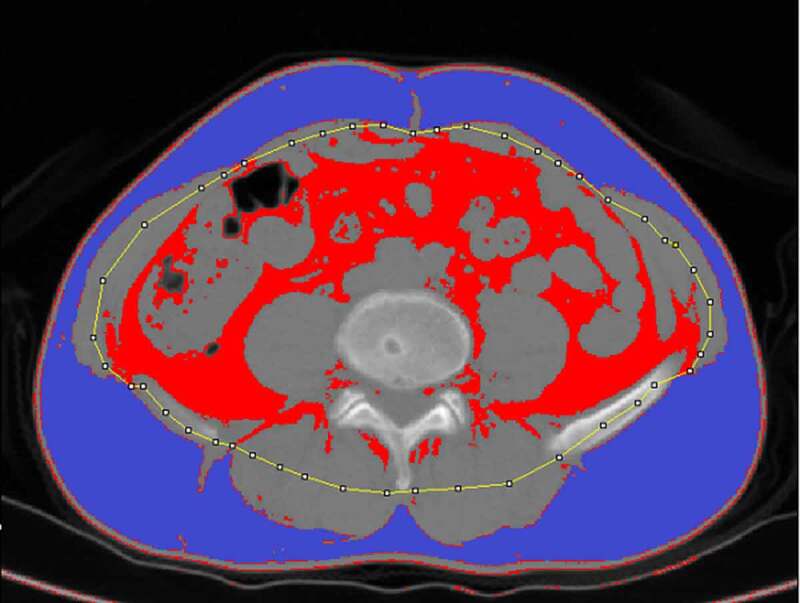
Fat measurements on CT. Subcutaneous fat (SFA, purple area) and Visceral fat (VFA, red area) were segmented after outlines (yellow line) were placed in the abdominal skeletal muscle from the original CT image for automatic calculation
